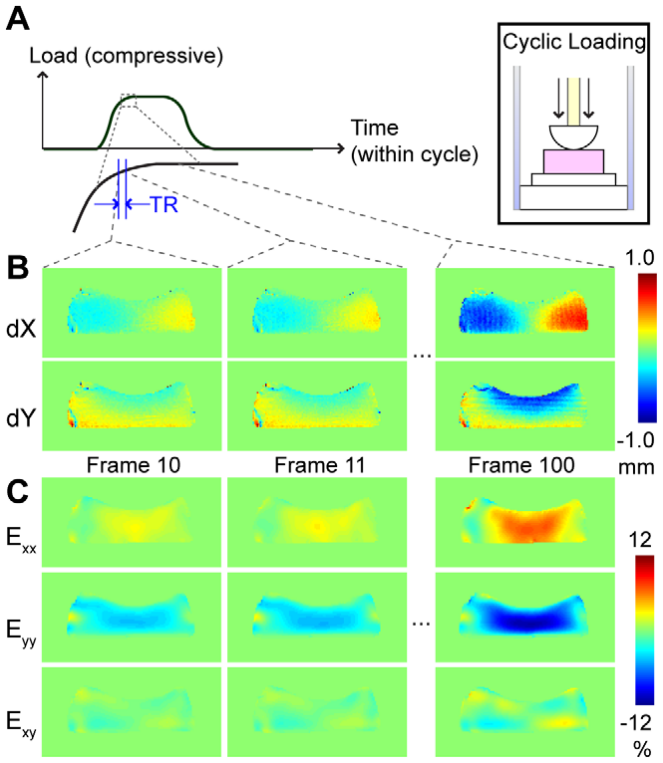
DENSE-FISP of transient mechanical behavior. High temporal resolution displacement-encoded MRI nondestructively measured displacements and strains at a sampling rate of 444 Hz (TR = 2.25 ms). Cyclic compression was applied to a silicone gel using a rounded indenter, and a 100-frame cine DENSE-FISP image was acquired during a transient portion of the loading curve (*A*). In-plane displacements (*B*) and strains (*C*) shown for frame numbers 10, 11, and 100 demonstrate that DENSE-FISP is capable of measuring transient mechanical behaviors at high temporal resolution (*B*).
